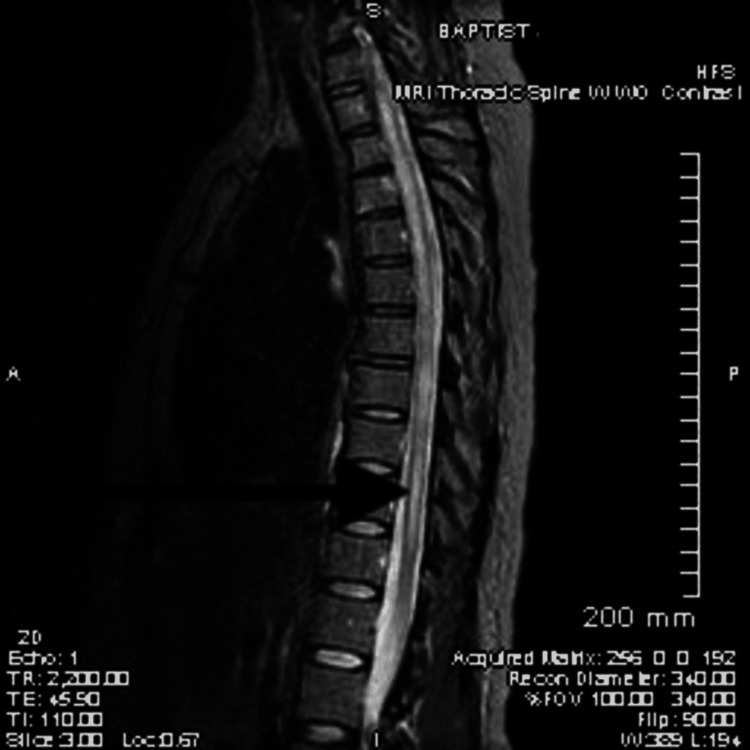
MRI thoracic spine with and without contrast shows diffuse abnormal central cord signal intensity throughout thoracic spine, extending into cervical cord and conus with focal enhancement in left central region at T7-T8 level.
